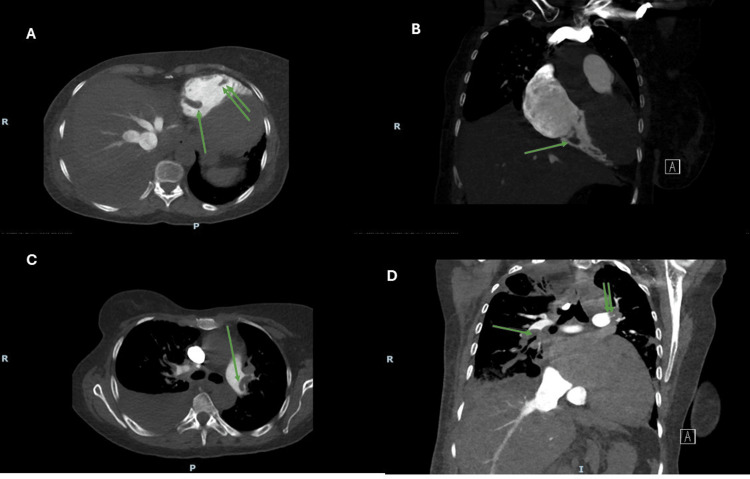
Case 1: Computed tomography pulmonary arteriography (CTPA). Panel A (axial view at the level of the right ventricle) showing the thrombus. Single green arrow and double green arrows pointing to the right ventricle thrombus in transit. Panel B (sagittal view) showing a thrombus in transit after passing through the tricuspid valve into the right ventricle. Panel C (axial view at the level of the pulmonary artery) showing a saddle-shaped embolus at the bifurcation of the left pulmonary artery and upper left lobe branch. Panel D (sagittal view at the level of pulmonary arteries). Single and double green arrows showing the continuation of the thrombi from the main pulmonary arteries into the lobar and inter-lobar branches bilaterally.
